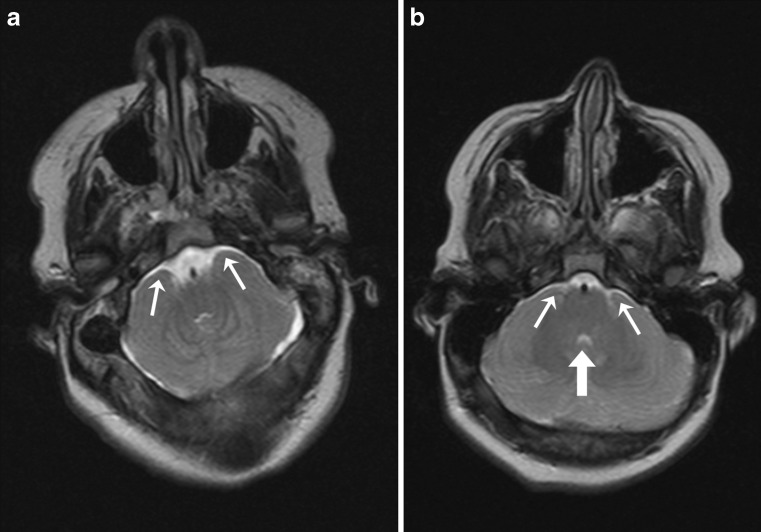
a Axial T2-weighted brain MR image in 16-year-old child with open spinal dysraphism. The image clearly shows that the cerebellar hemispheres are wrapped around the brainstem (*small white arrows*); b axial T2-weighted brain MR image in 12-year-old child with open spinal dysraphism. In this image, it is questionable whether the cerebellar hemispheres are wrapped around the brainstem (*small white arrows*). Also note the small fourth ventricle (*large white arrow*)
